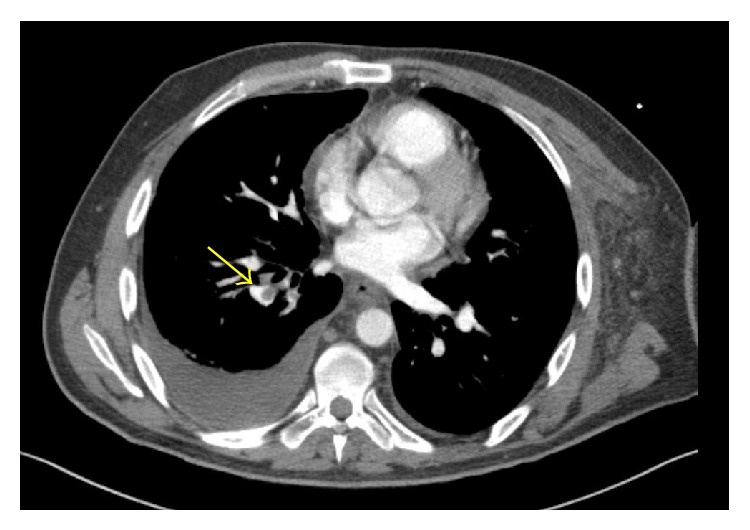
CT angiography demonstrating lobar and segmental pulmonary emboli (arrow) in the right lower lobe.
